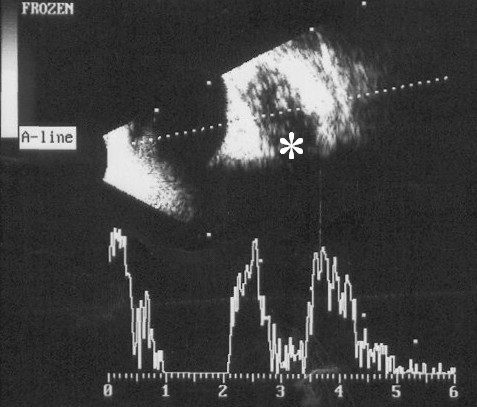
Orbital cellulites. High-resolution (10 MHz) ultrasonogram of right orbit showing an ill-demarcated hypoechoic collection (asterisk) with internal echoes, behind the globe. The scale bar at the bottom is in centimetres.
